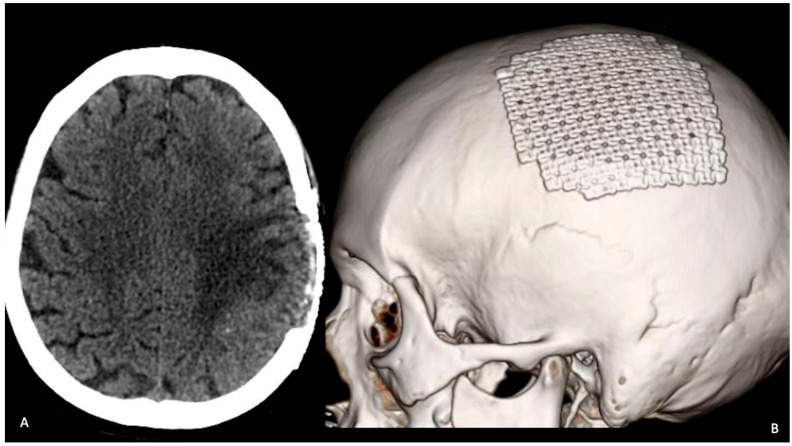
(A) CT axial scan performed 30 days after surgery showing good radiological outcome. (B) 3D detail of the mesh used for skull reconstruction.
